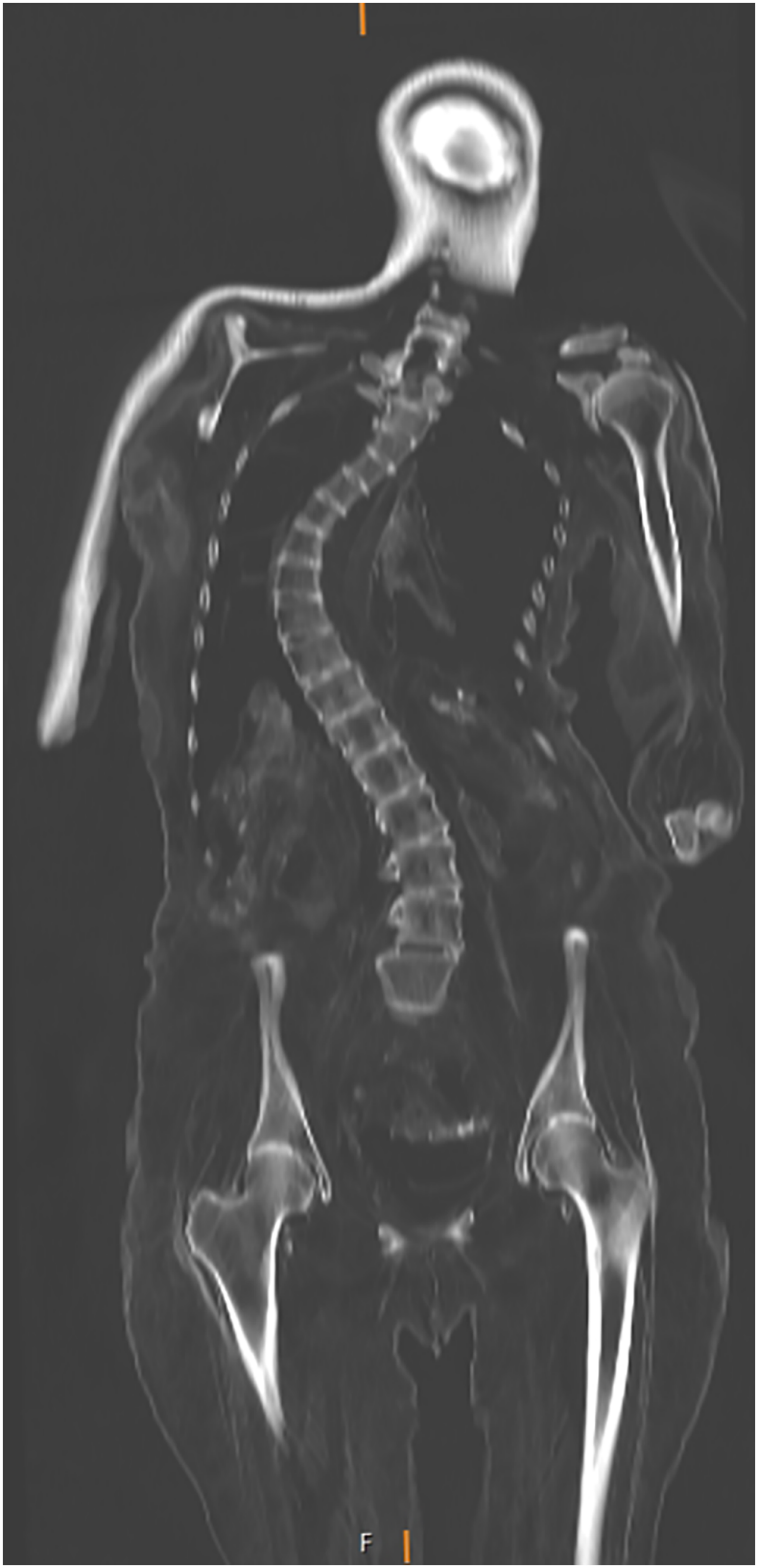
Coronal multi-planar reconstruction of a CT data set showing the spine of individual C with lateral deviation (scoliosis).
